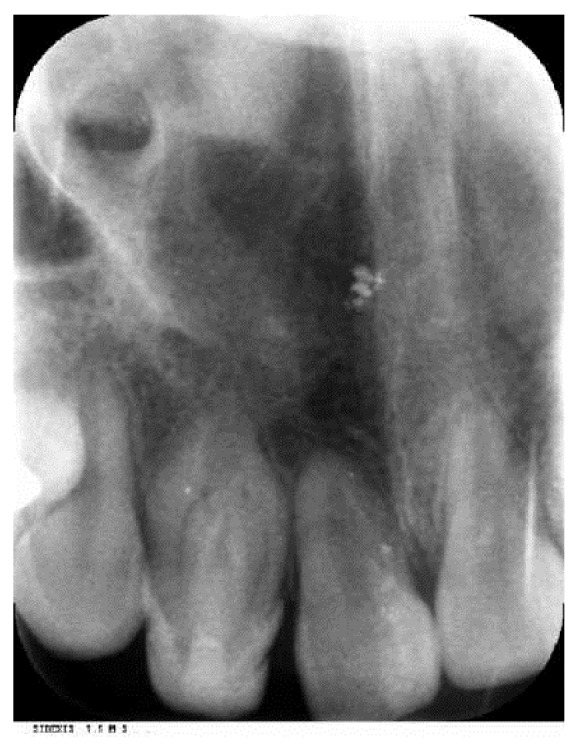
The twenty-month follow-up radiograph showing that the open apex was closed.
